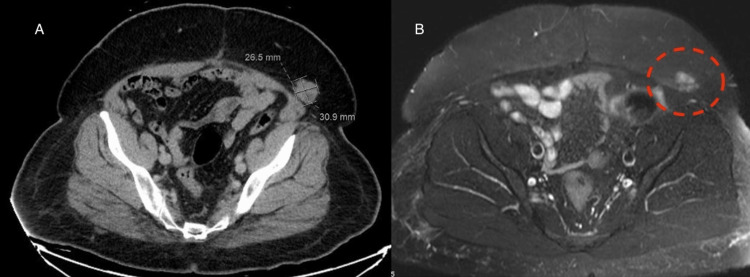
(A) CT scan of the pelvis showing a 2.6 x 3.1 cm mass located in the left groin area and (B) MRI showing left groin subcutaneous soft tissue lesion demonstrating intermediately low signal intensity on T2
